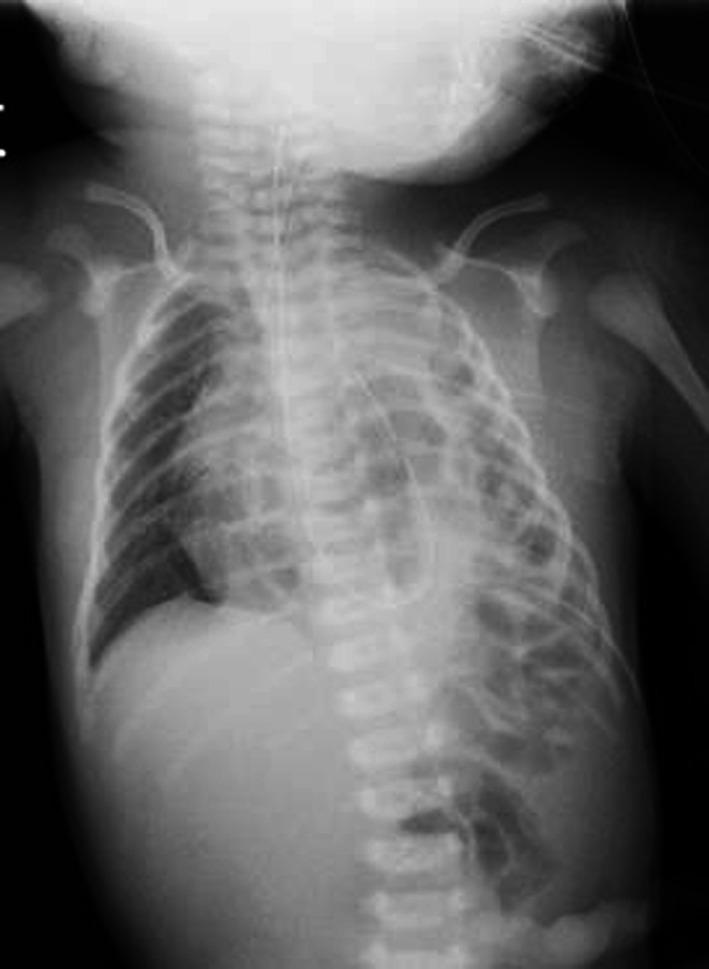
Chest X-ray shows the stomach and intestine in the thoracic cavity. The diagnosis of CDH was confirmed
